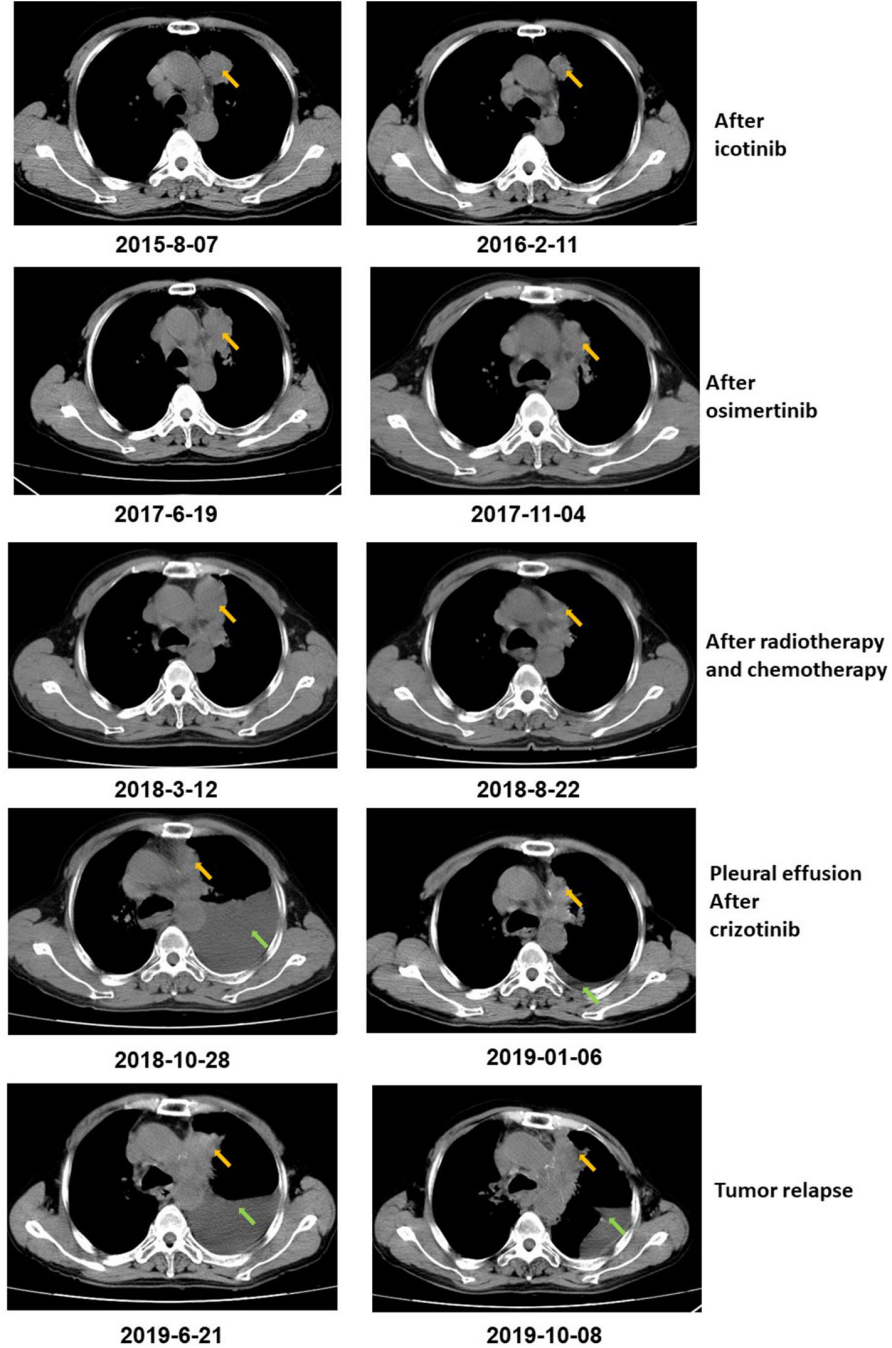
CT scan showed that the tumor size decreased after icotinib treatment, osimertinib treatment, and radiotherapy and chemotherapy, respectively. After icotinib treatment, the tumor size (yellow arrow) decreased from 39 mm*36 mm to 39 mm*27 mm in February 2016. After icotinib-resistance and osimertinib treatment, the tumor size (yellow arrow) decreased from 42 mm*34 mm to 40 mm*22 mm in November 2017. After radiotherapy and chemotherapy during March 2018 to June 2018, the tumor size decreased from 49 mm*32 mm to 41 mm*20 mm. After October 2018, pleural effusion (green arrows) was developed in the patient and extracted. The patient achieved a stable disease (SD) during crizotinib treatment in June 2019, compared with October 2018, with tumor mass measuring from 36 mm*30 mm to 36 mm*27 mm.
